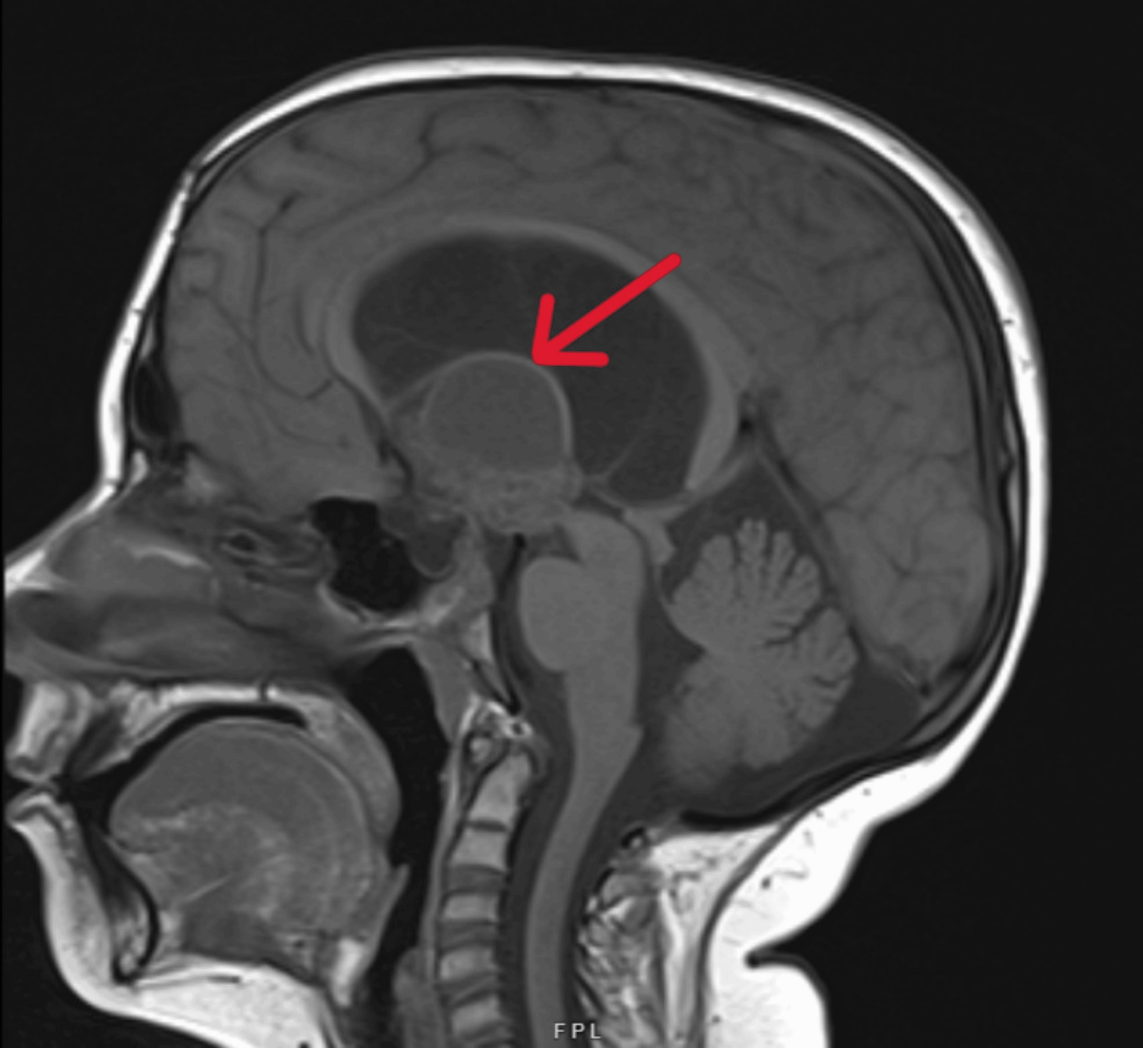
MRI brain with and without contrast, 2022 The arrow depicts craniopharyngioma comprising of multiple cystic components and a solid nodular enhancing component extending into the hypothalamus as well as along the third ventricle, causing compression of the foramen of Monro and hydrocephalus. The largest cystic component measured approximately 2.8 cm. The nodular enhancing lobular area along the inferior aspect of the mass measured 2.9 cm.
